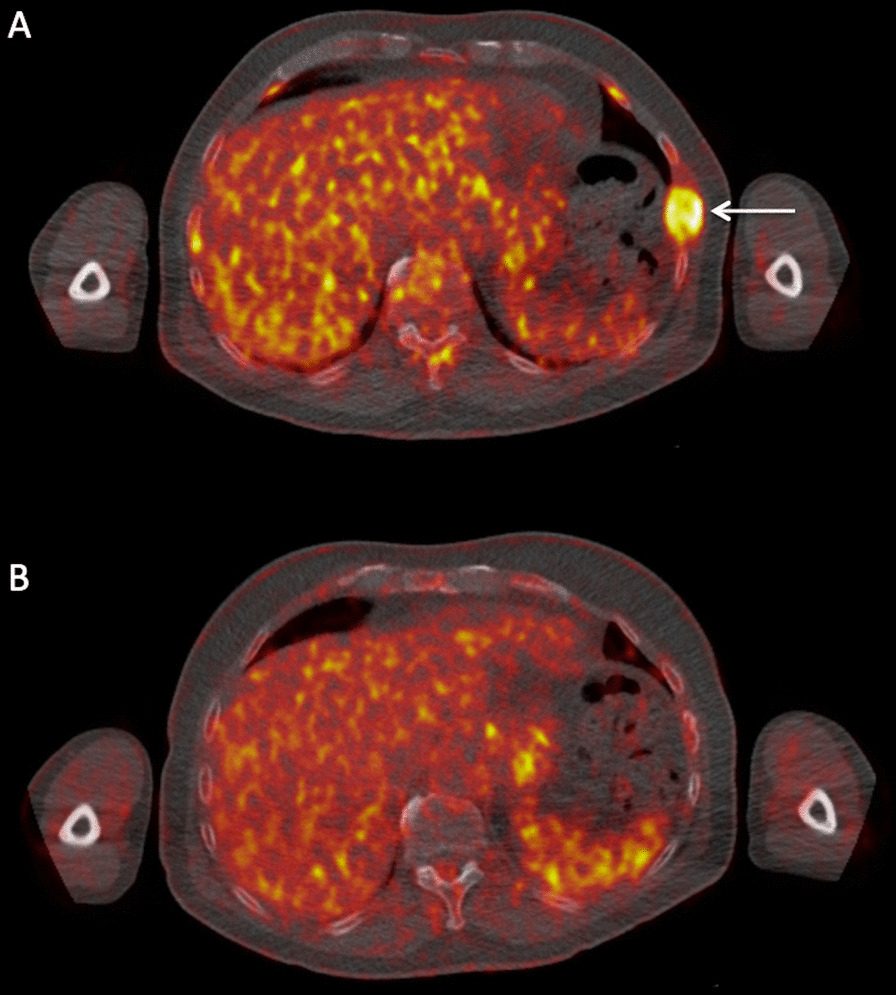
Transaxial PET/CT images at the thoracic level of a 70-year-old, male MM patient before and after therapy. A Baseline PET/CT revealed a hypermetabolic, focal lesion in the 8th left rib with cortical bone interruption and involvement of the surrounding soft tissues (arrow) as a sign of PMD. B After completion of HDT and ASCT, follow-up PET/CT revealed a complete metabolic remission of the former hypermetabolic PMD and development of a rib fracture
